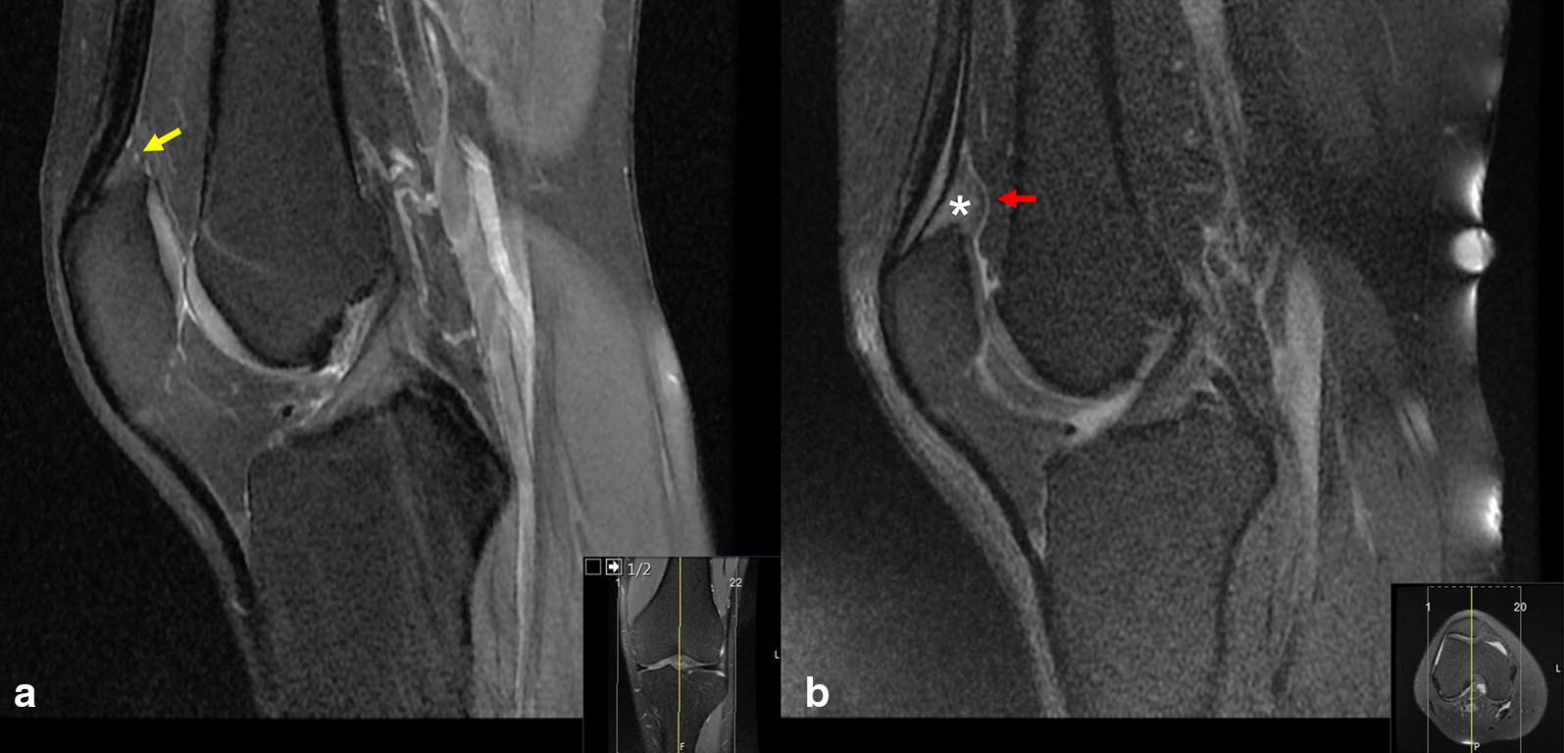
a Appearance of normal quadriceps fat pad (yellow arrow). b A patient with QPFIS. The sagittal fat-saturated, proton-density-weighted MRI demonstrates typical convex appearance (red arrow) and increased signal and thickening within quadriceps fat pad (white star)
